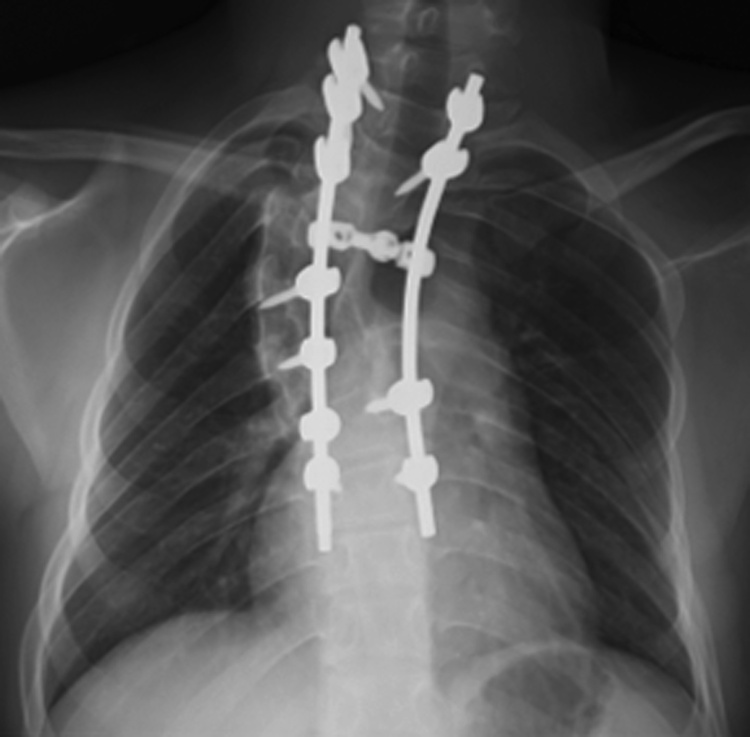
Postoperative plain X-ray showing improvement in the scoliosis.
